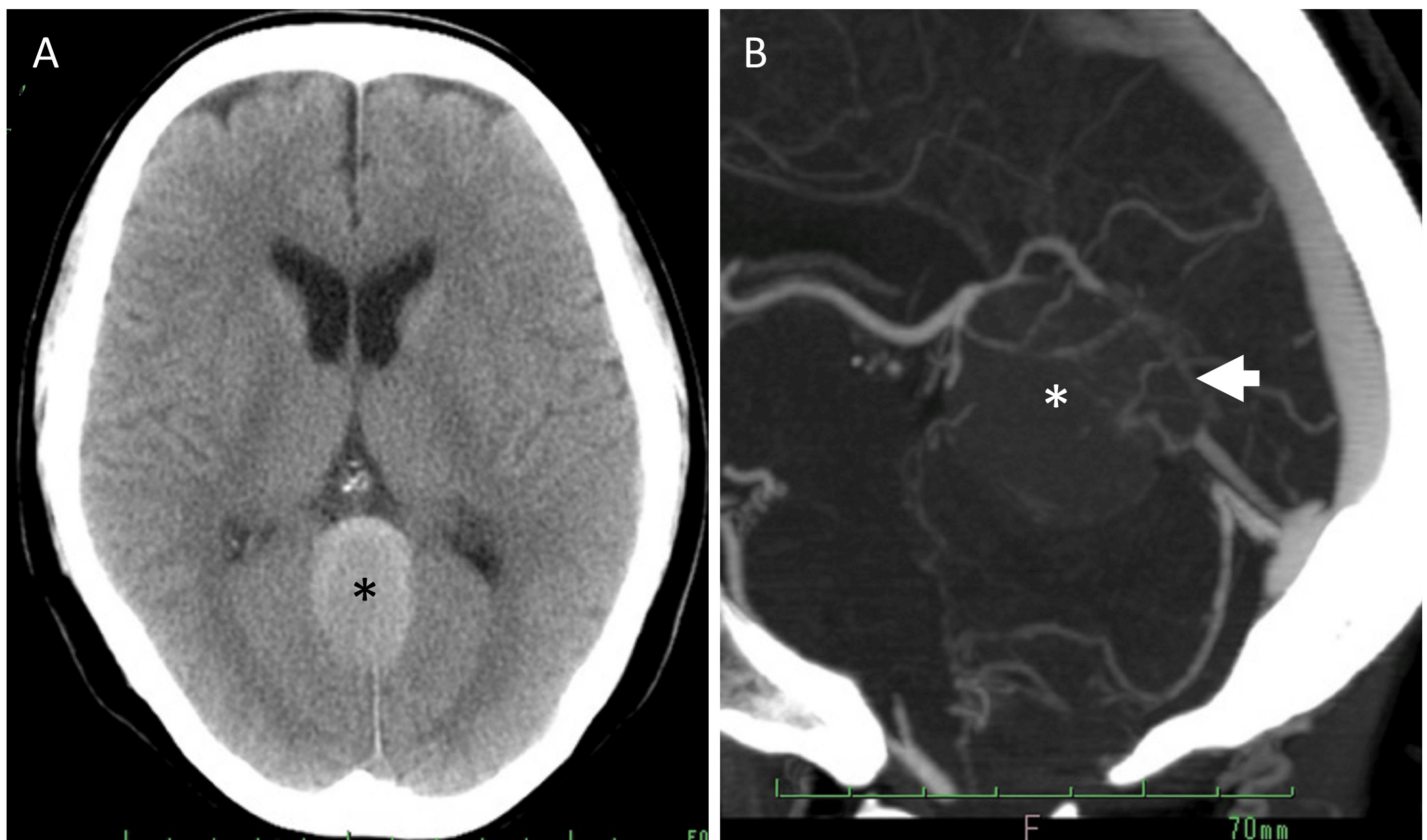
Preoperative computed tomography (CT) images (A) is a plain CT scan, and (B) is CT angiography. In (A), a falcotentorial meningioma (black asterisk) presenting as a hyperdense area is observed, although no hydrocephalus is seen. In (B), the tumor (white asterisk) shows minimal enhancement, and the straight sinus (arrow) is not visualized.
